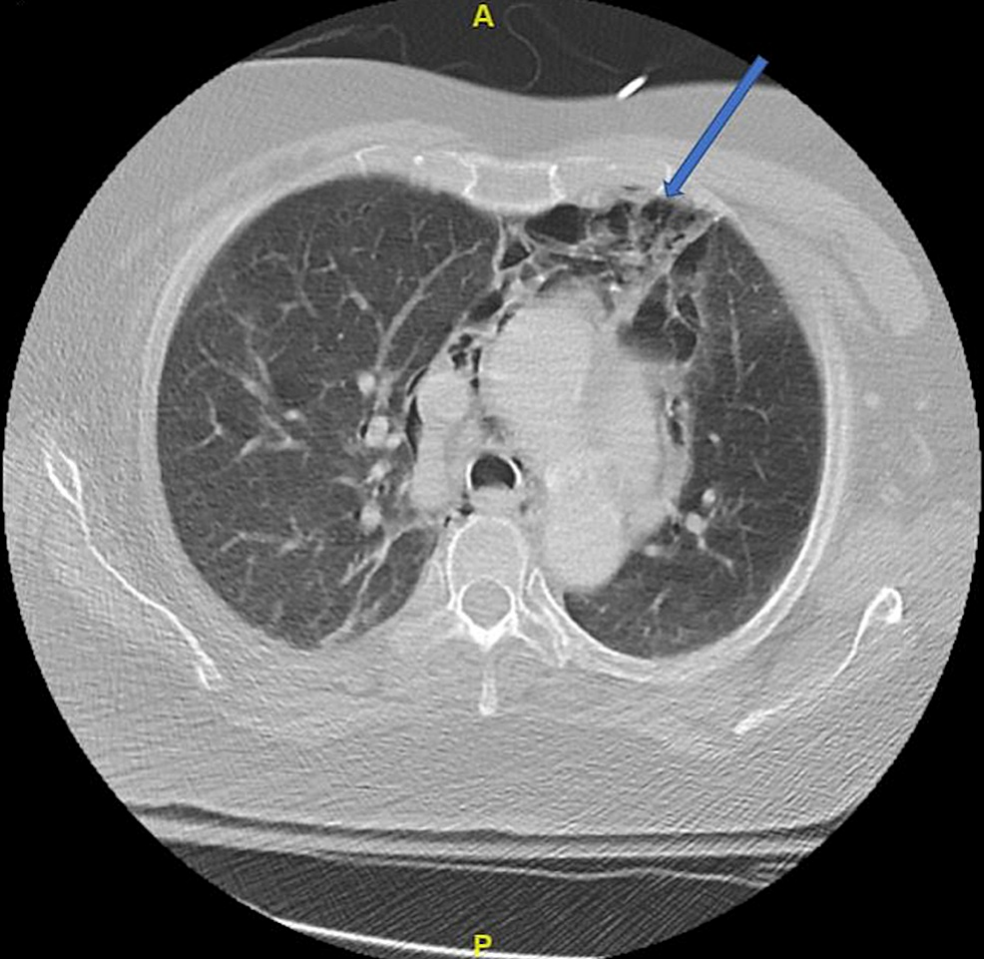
Computed tomography chest showing pneumomediastinum (blue arrow)
